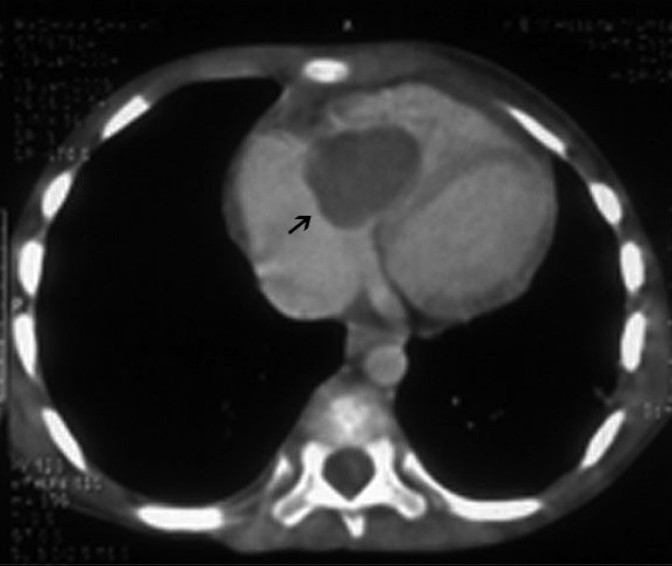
Cardiac CT scan picture showing the vegetation in right ventricle (arrow)
